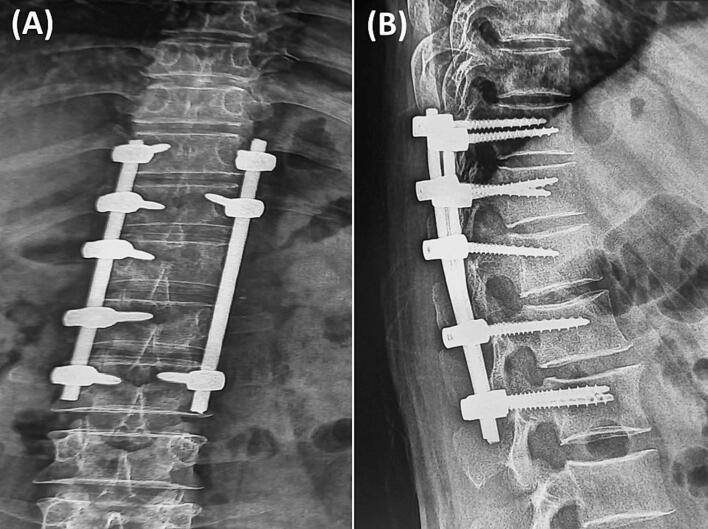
Postoperative thoracolumbar radiographs: (A) anteroposterior and (B) lateral views showing pedicle screw–rod fixation from D10 to L2 for stabilization following hydatid cyst excision.
